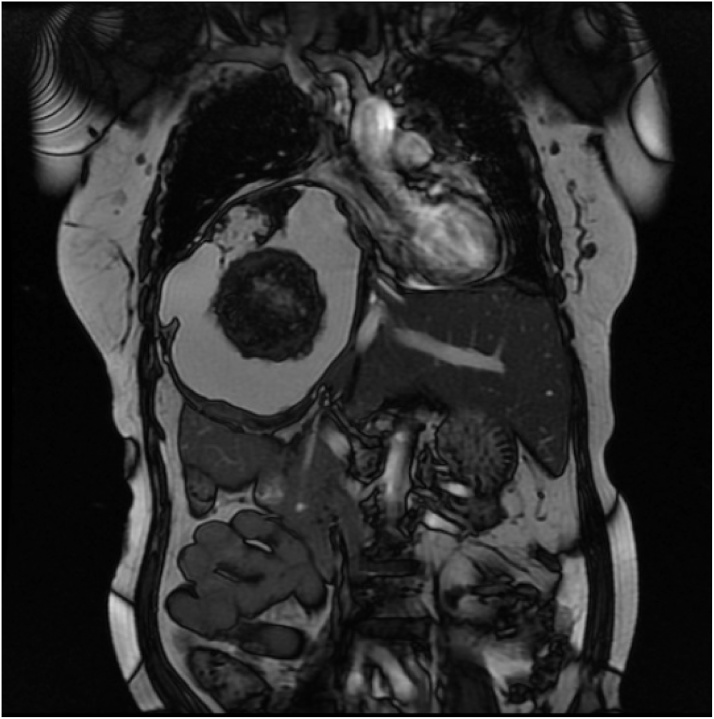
MRI Abdomen/Pelvis Coronal section of right upper quadrant mass demonstrating mass effect and marked upward displacement of right diaphragm.
